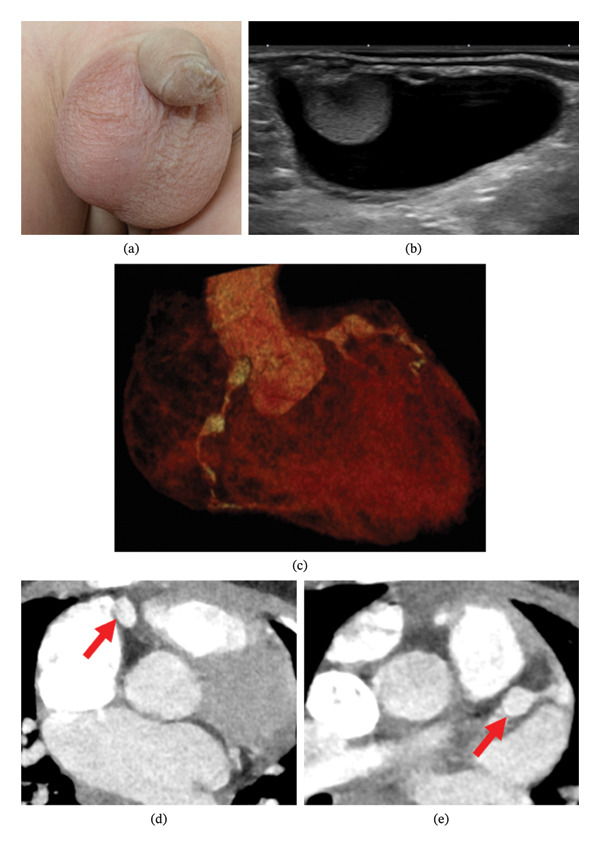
Clinical and imaging findings in the acute phase of Kawasaki disease. (a) Photograph of the scrotum on illness day 9 showing erythema and swelling localized to the right side. (b) Scrotal ultrasonography demonstrating a right‐sided hydrocele. (c) Coronary CT providing an overall view of the coronary arteries and revealing aneurysmal changes in the RCA, LMT, LAD, and LCX. (d) Coronary CT highlighting a coronary artery aneurysm of the RCA (red arrow indicates the aneurysm). (e) Coronary CT highlighting a coronary artery aneurysm of the LAD (red arrow indicates the aneurysm). CT, computed tomography; RCA, right coronary artery; LMT, left main trunk; LAD, left anterior descending artery; LCX, left circumflex artery.
